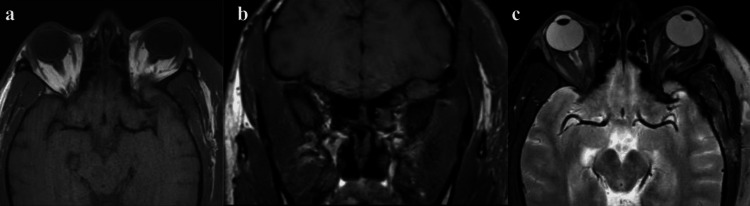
Postoperative MRI demonstrating no residual mass (a, b) Axial and coronal T1-weighted MR images showing no evidence for residual mass in the greater wing of the left sphenoid bone status post resection. (c) Axial T2-weighted MR image status post resection with no residual signal changes.
